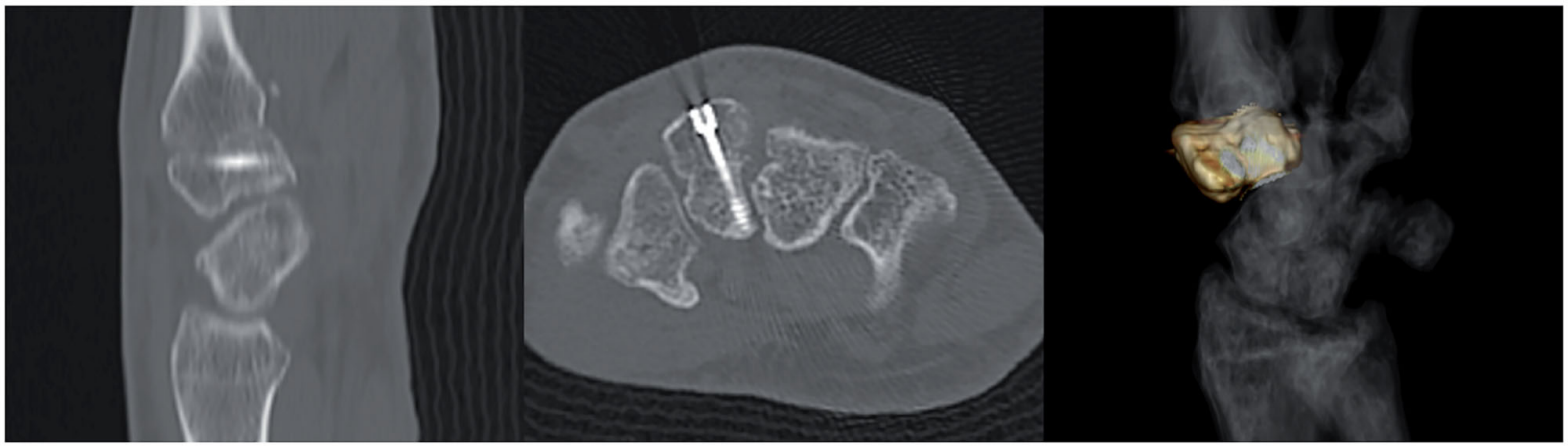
Postoperative computed tomography images obtained at one year after surgical fixation of an isolated left trapezoid coronal shearing fracture in a 40-year-old male. Complete bone union of the trapezoid is visible without any arthritic changes of the second carpometacarpal joint or necrosis of trapezoid. The headless compression screw does not protrude out of the bone. There is no step in the joint and no separation at the fracture site.
